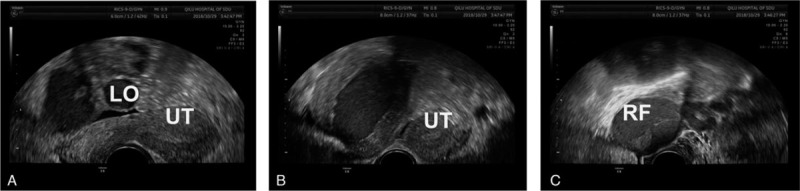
Ultrasound showing uterine dysplasia (right rudimentary uterine horn and left unicornate uterus) and hematometra of the reported case. A: left unicornate uterus and ovary (UT: uterus, LO: left ovary). B: hematometra in right rudimentary uterine horn. C: expansion of the right fallopian tube and ovary (RF: right fallopian tube).
